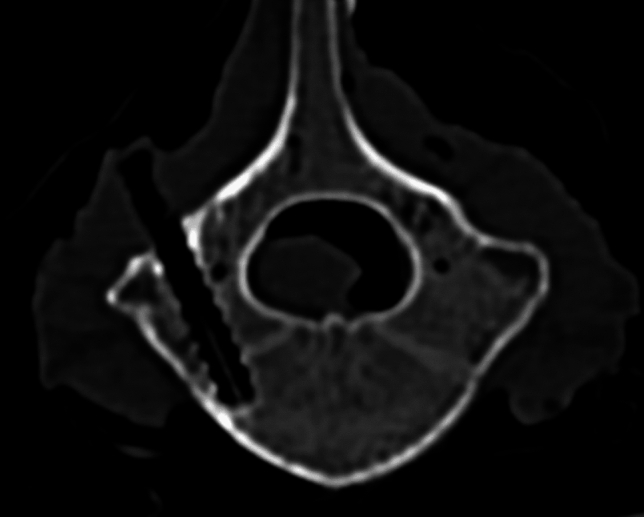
Computed tomography (CT) scan showing the threaded portion of a partially threaded spinal epiphysiodesis trajectory (SET) screw inadvertently traversing the neurocentral synchondrosis (NCS)
